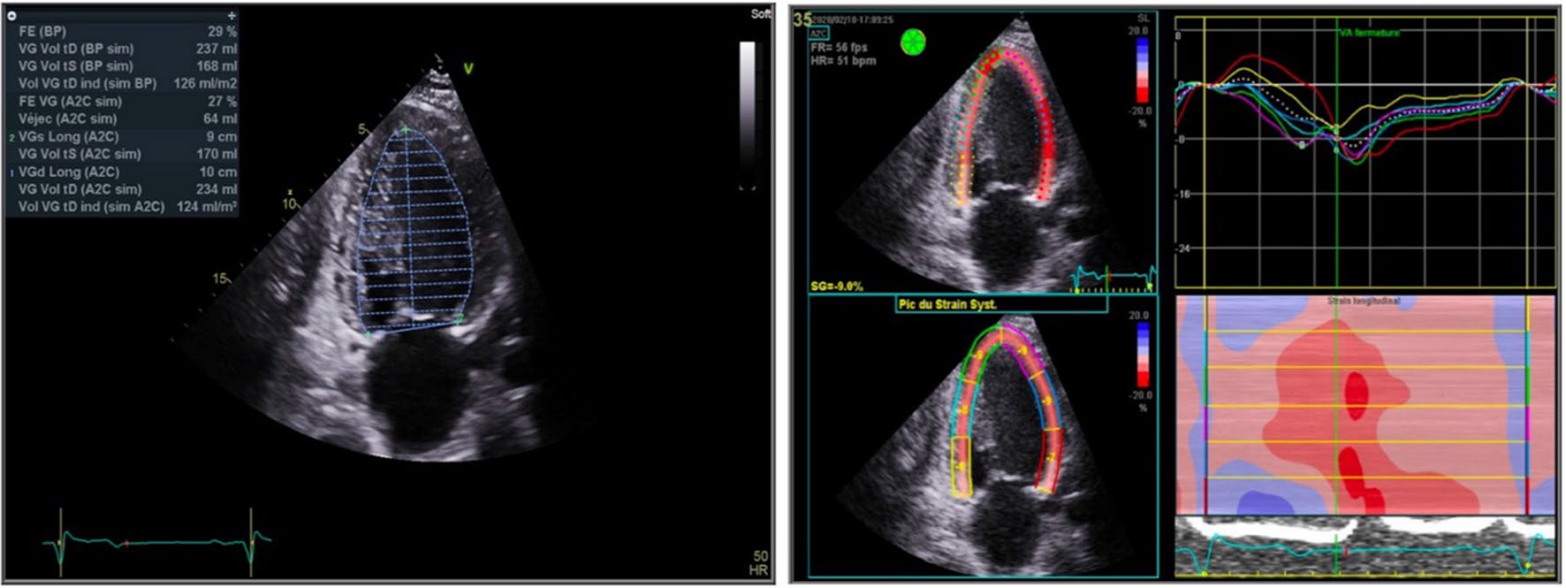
Transthoracic echocardiography shows LV parameters (left) and LV strain (right)
